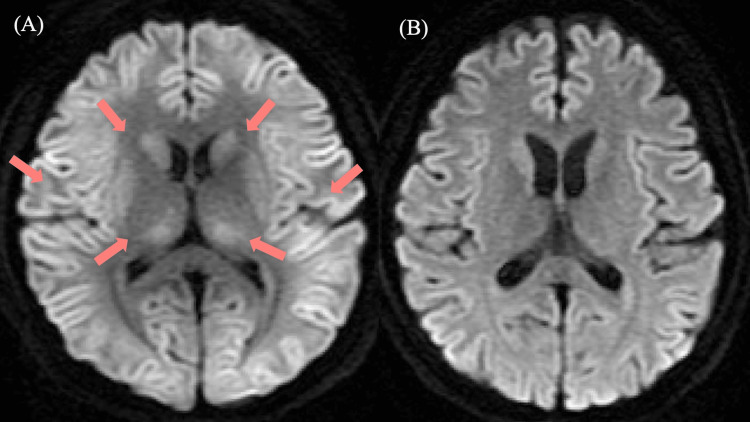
Brain magnetic resonance imaging (MRI) on days 5 and 11 of admission. (A) Axial diffusion-weighted imaging (DWI; b = 1000 s/mm^2^) on day 5 showing diffuse high-intensity signals in the bilateral cerebral cortex and thalami (arrows), consistent with toxic-metabolic encephalopathy. (B) Axial DWI (b = 1000 s/mm^2^) on day 11 demonstrating resolution of the previously observed abnormalities.
